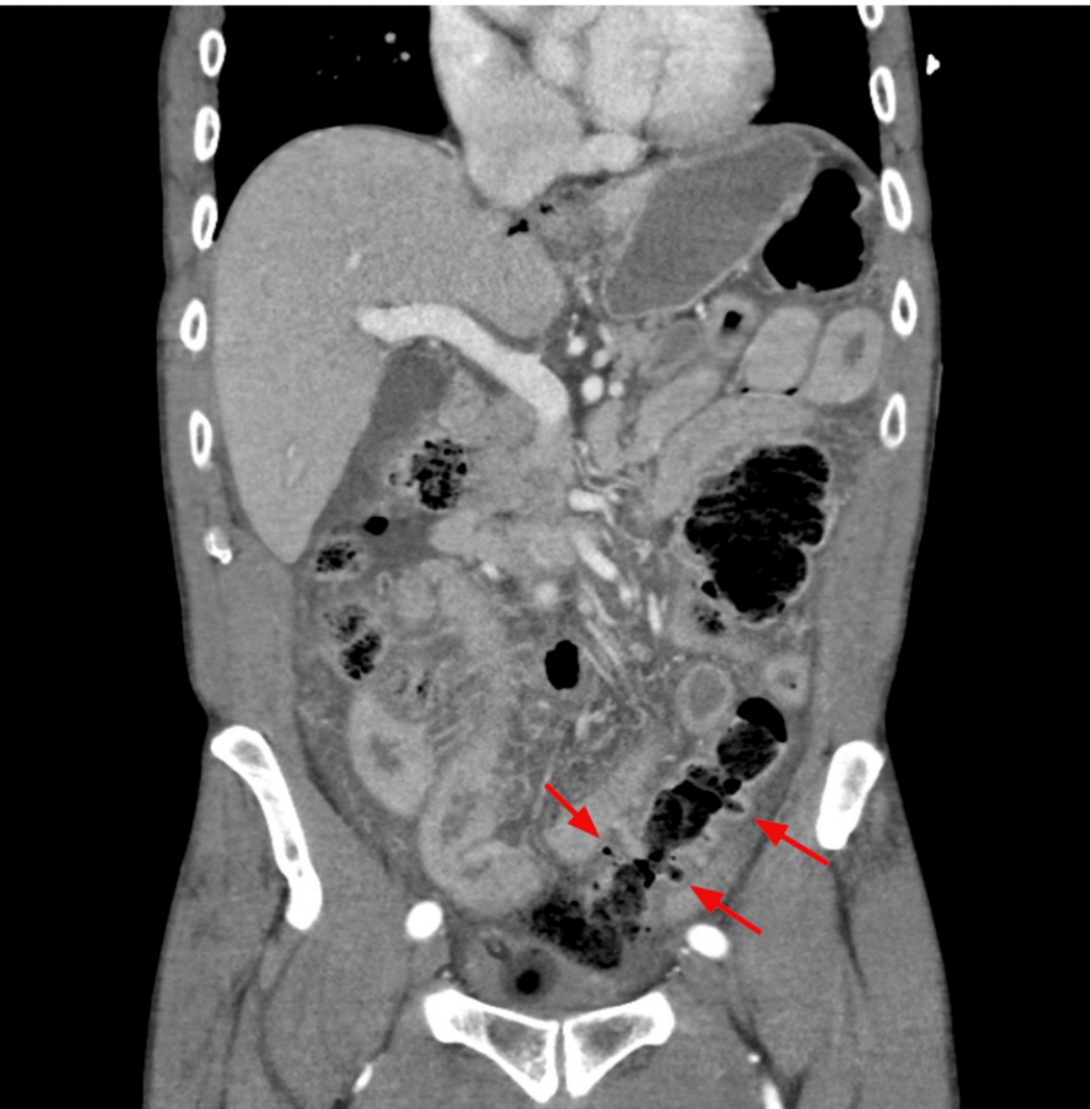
CT in the coronal view showing extensive colonic diverticular disease (red arrows)
